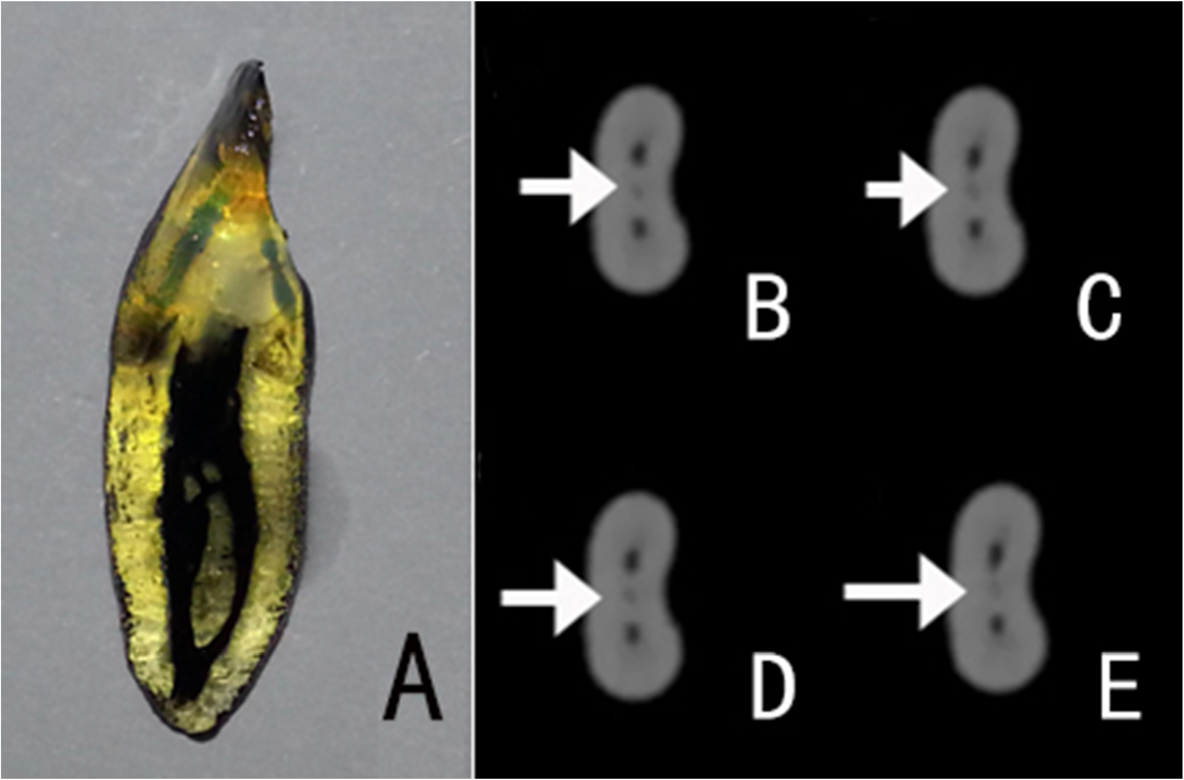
Image of a tooth with inter-canal communications. The white arrows denote the inter-canal communication. a Cleared tooth demonstrating inter-canal communications. b–e Continuous CBCT images in the axial view: A tiny low-density canal can be seen migrating between the two canals
